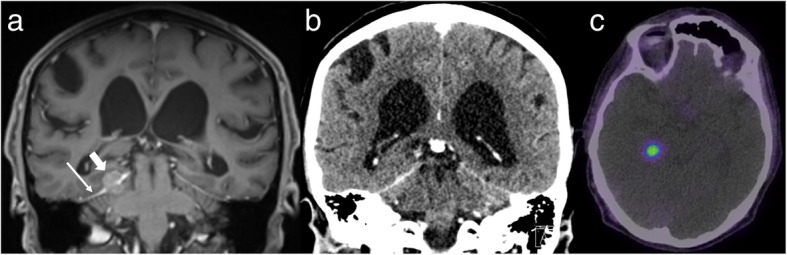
Routine post-contrast MRI surveillance (a) for a patient with metastatic melanoma demonstrates a durally-based mass related to the right side of the tentorium cerebelli, best seen in the coronal plane (short arrow). The appearances are suggestive of a meningioma, but the lesion was much smaller on a CT performed only 8 months earlier (b), raising concern for a metastasis. Further characterisation with GaTate-PET (c) demonstrates high uptake, confirming the diagnosis of a meningioma rather than a metastasis
